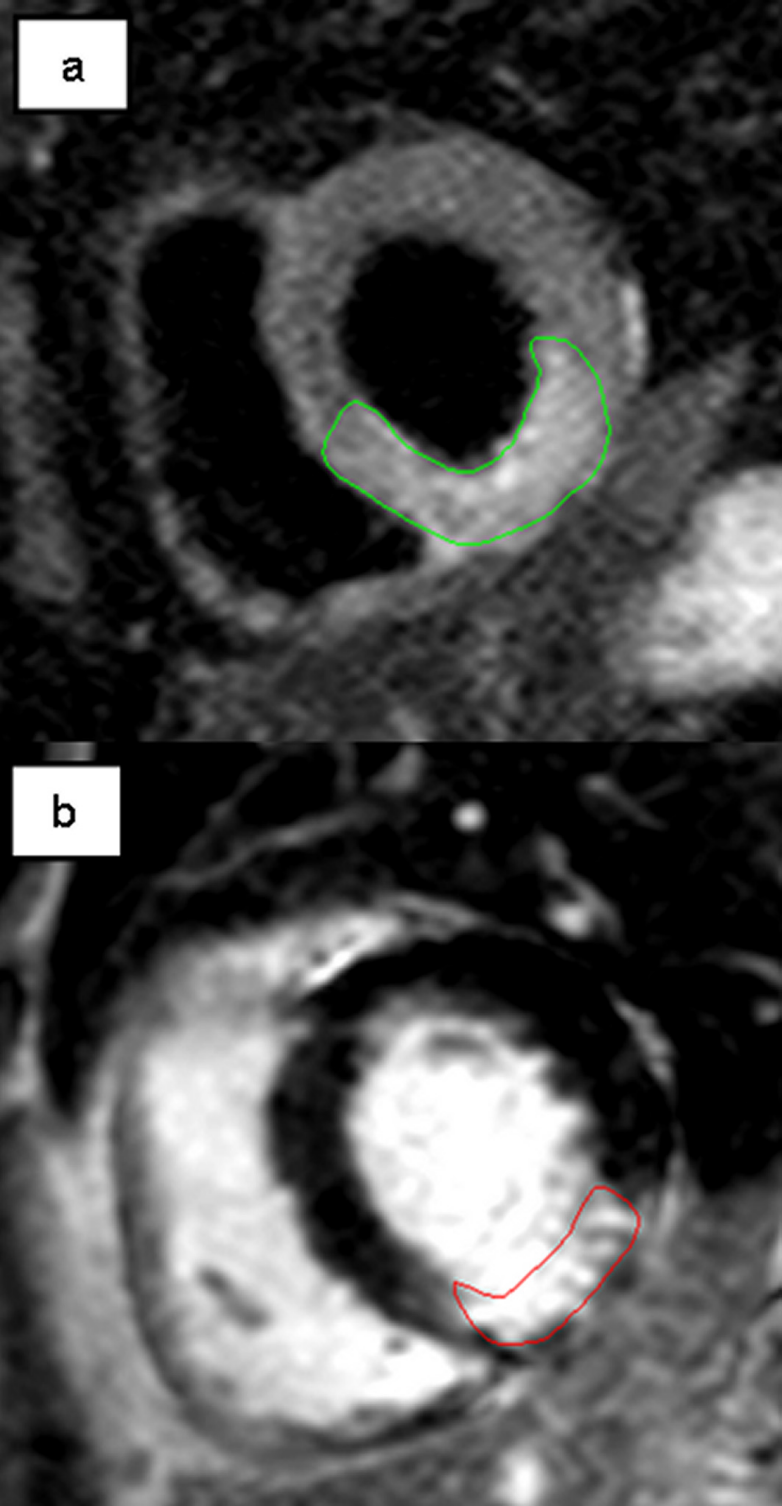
Patient with inferior/inferolateral STEMI due to subtotal stenosis of right coronary artery. a. T2-weighted image acquisition for the detection of myocardial edema (green contours), corresponding to the area at risk. b. Late enhancement CMR imaging showing infarcted area (red contours). Myocardial salvage can be calculated by comparing the area at risk in T2-weighted and infarct size in late enhancement images.
